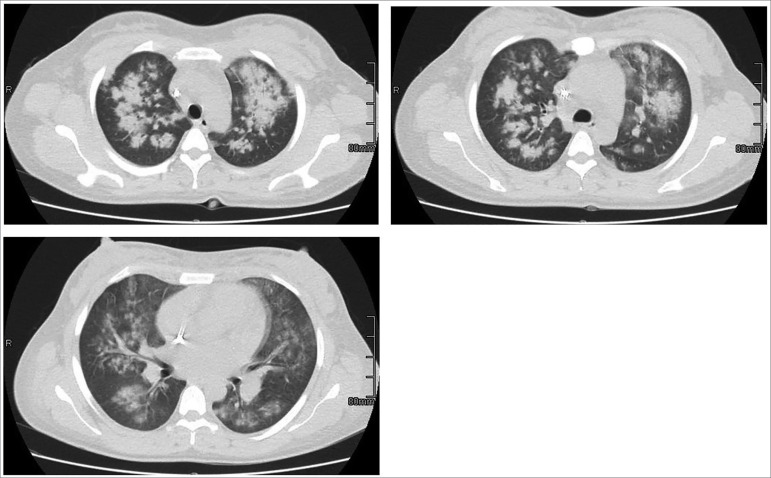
Axial cut in the the pulmonary window of high-resolution chest computed tomography. It is note extensive central alveolar occupation opacities located in both lung fields, with bilateral basal ground-glass areas in relation to multi-lobular consolidation compromise.
